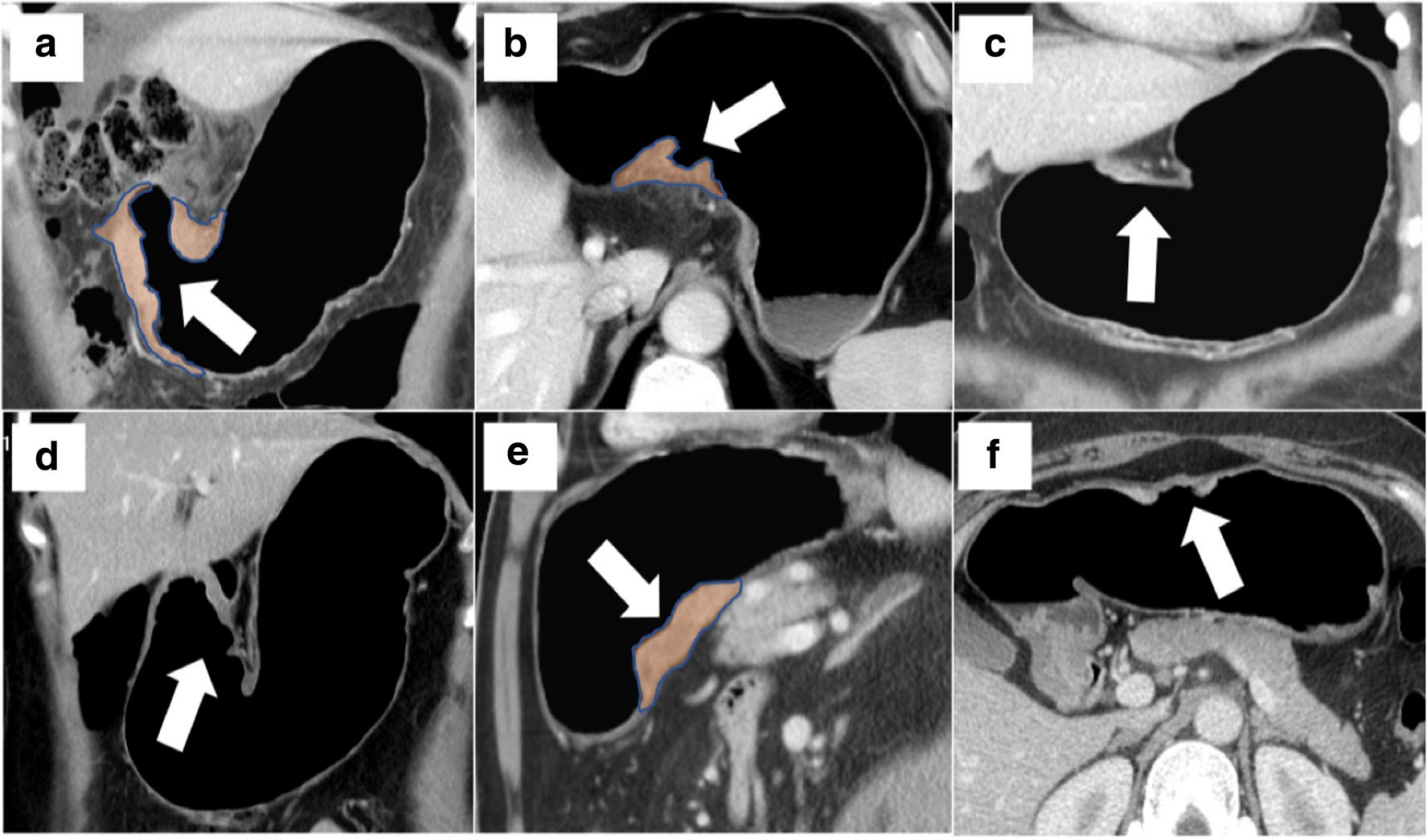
Contrast-enhanced multidetector computed tomography images of different gastric cancers (arrows) that have been acquired using a standard protocol, thus allowing accurate regions of interest for texture analysis (a, b, e, taken as an example)
